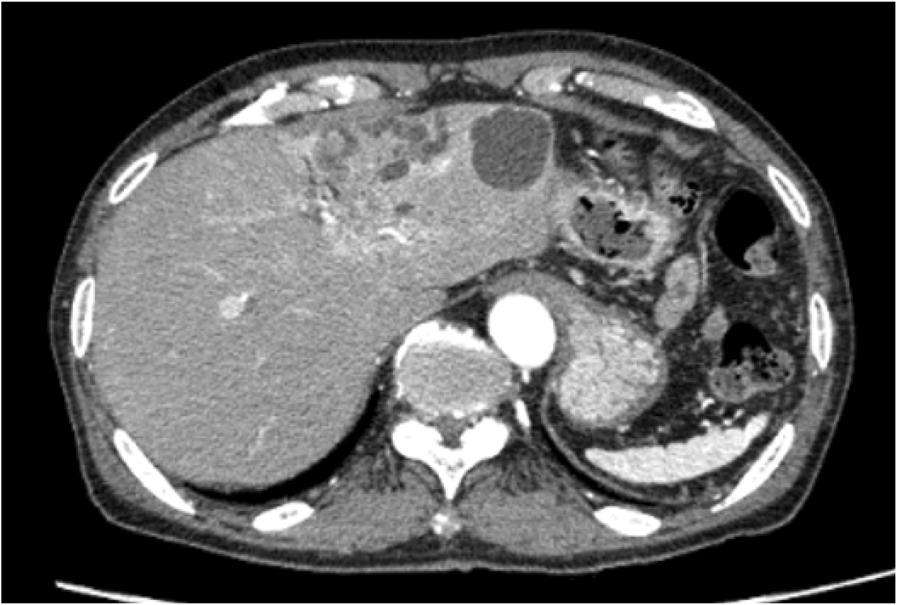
Computed tomography images showing a tumor in segment 3 (yellow arrow). The peripheral bile duct branch of segment 3 was dilated
